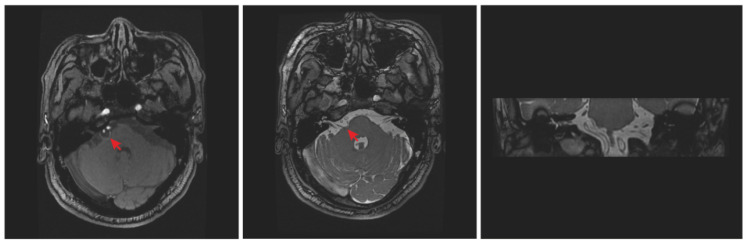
Preoperative MRTA showed that a cluster of cerebellar arteries compressed the root of the right facial nerve, but no sign of tumors was found (red arrowhead, left panel). The corresponding COSMIC sequence MRI at the same level was also provided (middle panel: axial section; right panel: coronal section).
